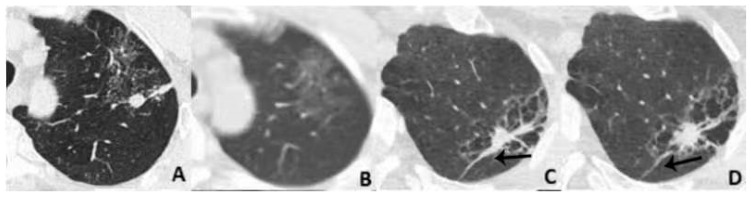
SNs reclassified by Modified Lung-RADS 2022. (A,B) Male, 77y, previous sigmoid colon cancer; a new SN of about 7.4 mm was detected in the upper lobe of the left lung (A), with a clear nodule boundary and cord around it. Patchy exudative lesions were significantly absorbed compared to a CT image from 6 months ago (B). The scores of Lung-RADS 2022 and Modified Lung-RADS 2022 were 4A and 2, respectively. Finally, the pathological diagnosis was tuberculoma. (C,D), male, 77y, prior thyroid carcinoma; a lesion with a size of 16.0 mm in the left upper lobe was found 11 days ago. Spiculation, lobulation, and pleural indentation around the nodule could be seen, and additionally, there were cord shadows (arrow) on the largest (D) and upper layers (C) of the nodule. The categories of the original version and Modified version were both 4X. Invasive adenocarcinoma was confirmed by pathology.
